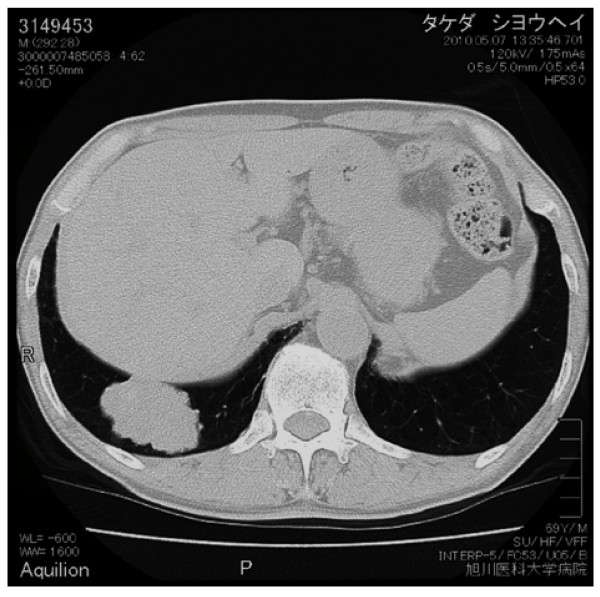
Chest CT revealed a lobulated mass measuring 65 mm in diameter involving S9 and S10 of the right lung
